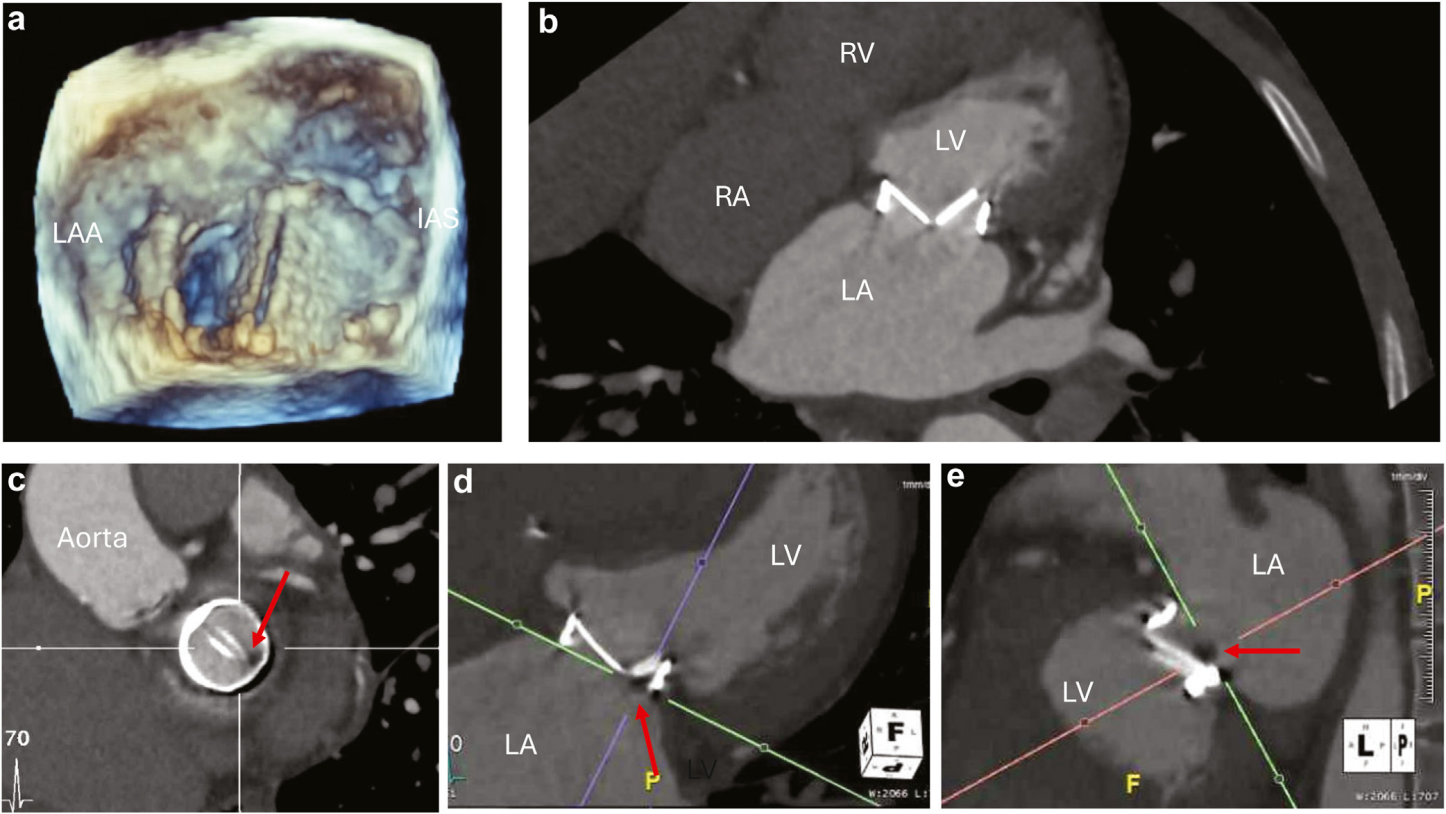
Patient with a mechanical valve in the mitral position with stenosis from a fixed medial occluder secondary to thrombus. (a) Three-dimensional (3D) transesophageal echocardiographic *en*-*face* view of a mechanical valve in the mitral position during diastole with a fixed medial occluder. The lateral occluder opens normally. (b) Cardiac computed tomography with contrast demonstrates the same findings. (c to e) Cardiac computed tomography multiplanar reconstructed views demonstrate the presence of a mass (red arrow) on the atrial side at the junction of the valve discs. The mass measured up to 5 mm and had low attenuation of 80-90 Hounsfield units, which is consistent with thrombus. Abbreviations: LA, left atrium; LAA, left atrial appendage; LV, left ventricle; RA, right atrium; RV, right ventricle.
